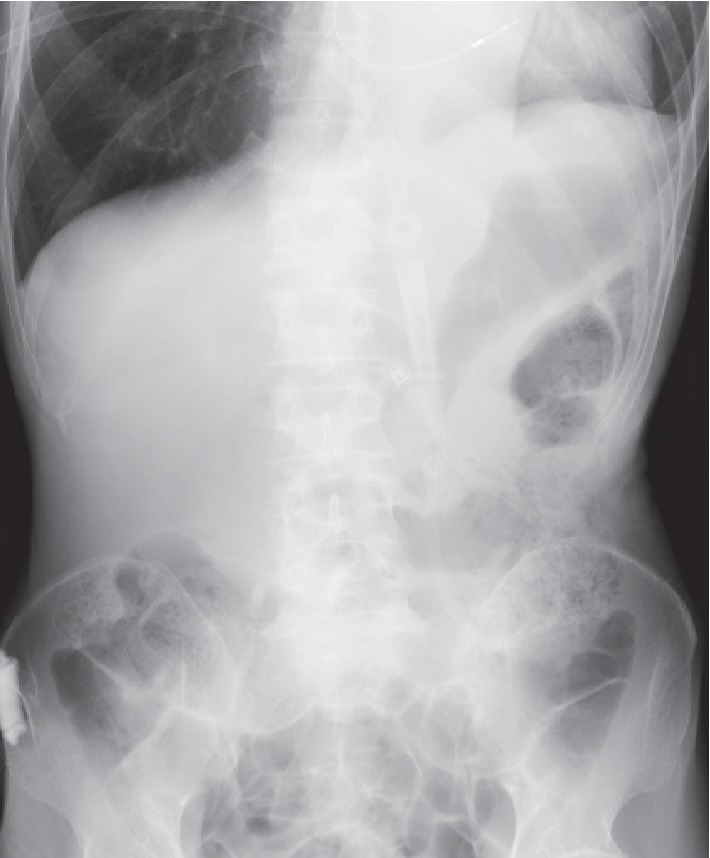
The X-ray image does not show a distended stomach.
